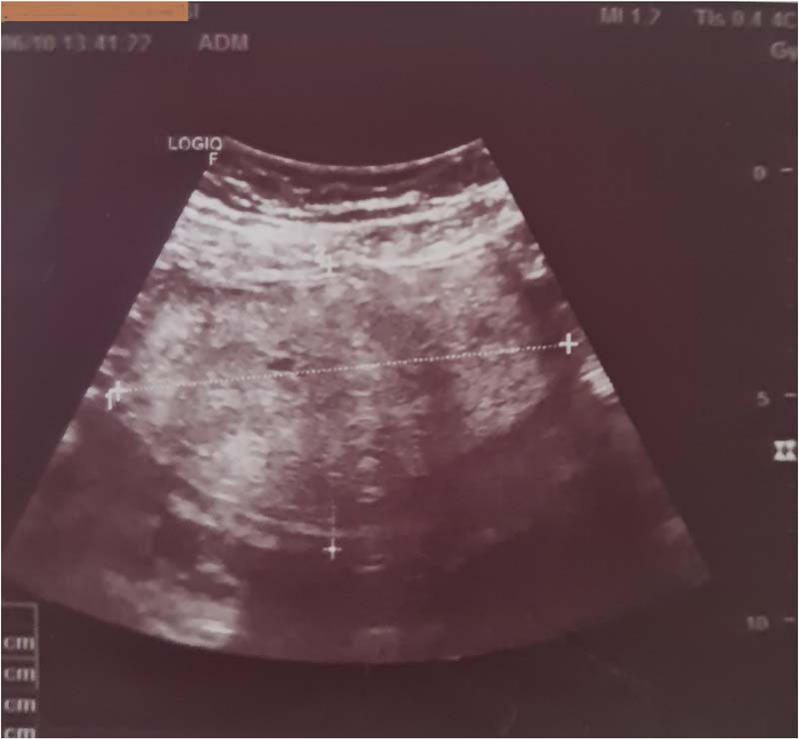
Ultrasound showing an oval, well-limited left ovarian mass, with regular contours, and a heterogeneous, hyperechoic echostructure and cystic areas.
